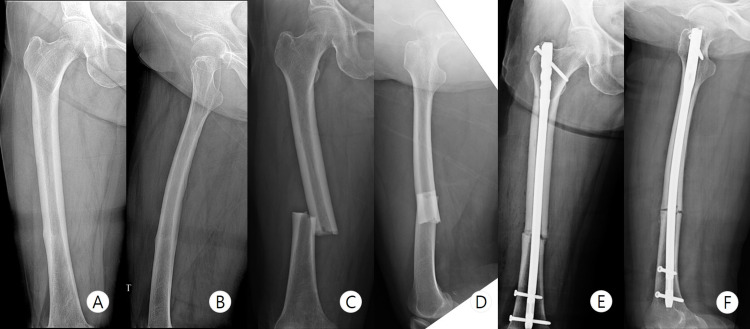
Previous imaging - case 2 Right femur anteroposterior (A) and lateral (B) radiographs 10 months before the complete fracture show lateral cortical hypertrophy, and the radiolucent line indicates incomplete fracture. Complete fracture of the right femur (C, D) and immediate postoperative X-ray (E, F)
